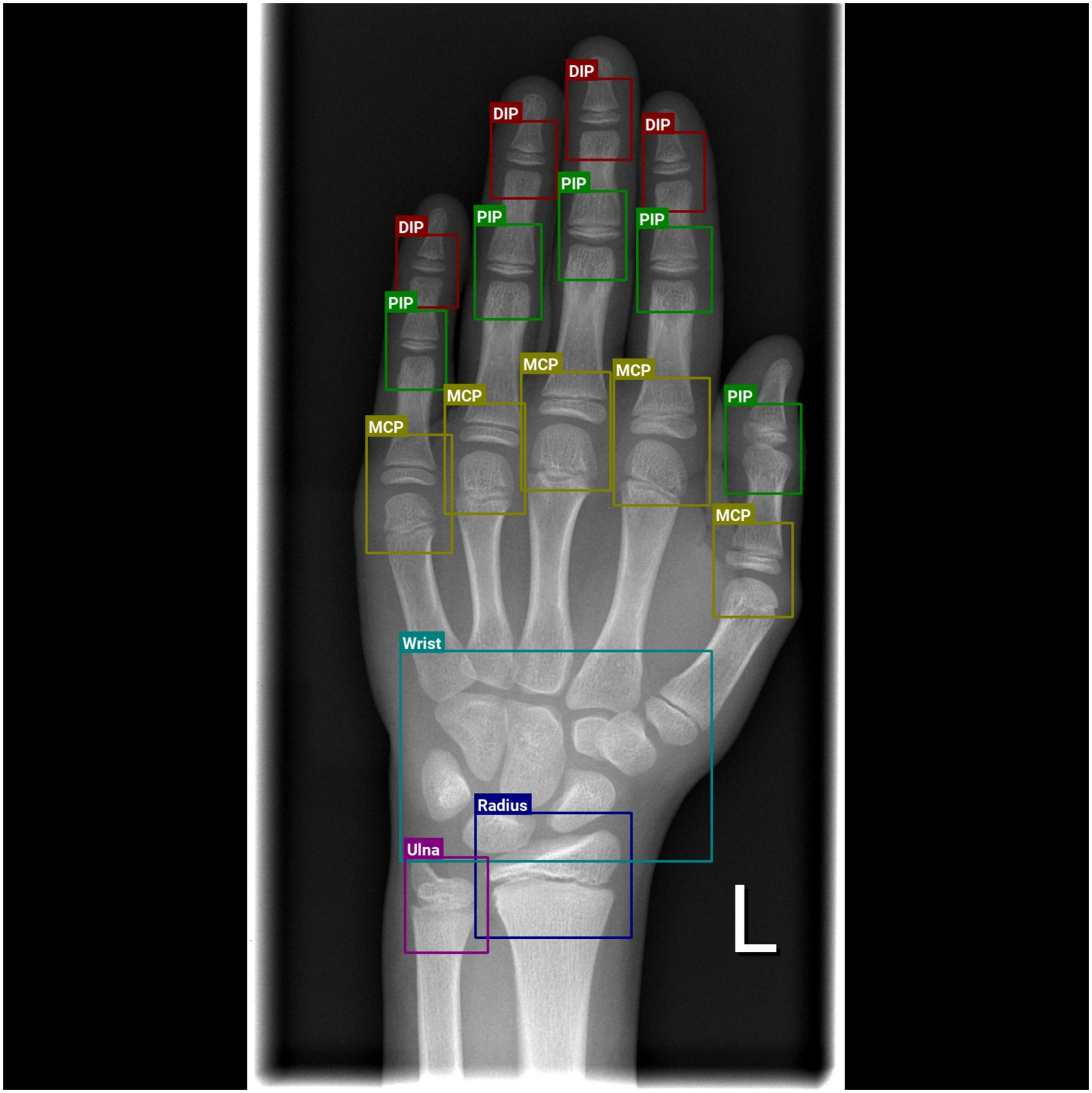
Example inference for a radiograph, highlighting all regions of interest using the final trained Faster-RCNN network.
